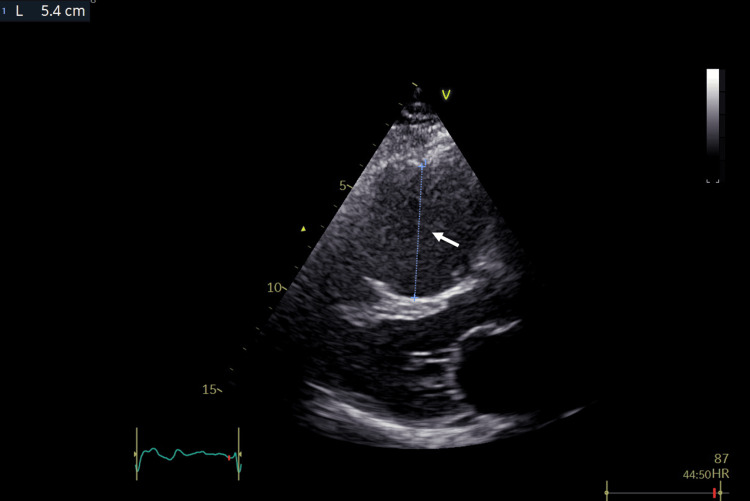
Transthoracic echocardiography (parasternal long-axis view) of Case 2 showing marked right ventricular dilation with an anteroposterior diameter of 54 mm (white arrow)
